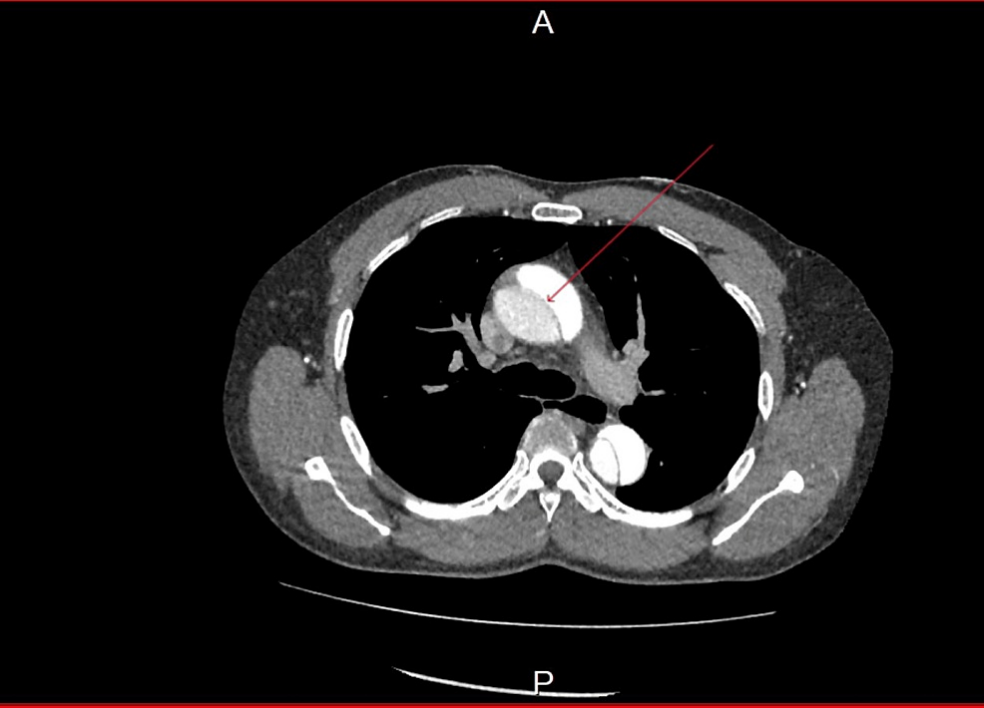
A CT angiogram of the aorta shows dissection of the ascending aorta.
